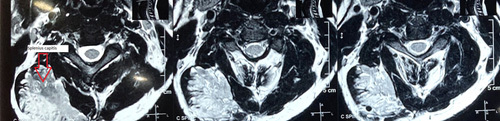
MRI of the cervical spine; T2-weighted scan (axial section) showing intramuscular hemangioma of splenius capitis (red arrow) with no extension to the adjacent muscle.
